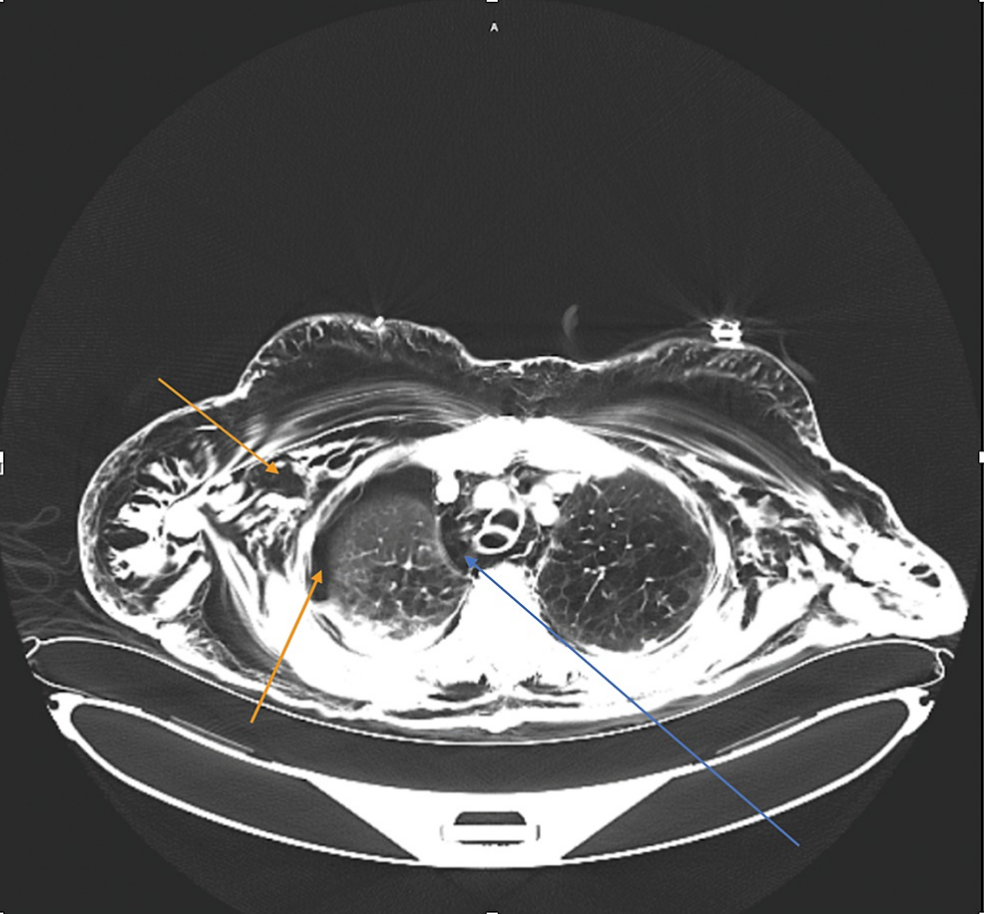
Computed tomography (CT) abdomen/pelvis (A/P) with intravenous contrast - axial view CT A/P in the regular window. Evident is the presence of extensive subcutaneous emphysema (yellow arrow), pneumomediastinum (blue arrow), and a right-sided pneumothorax (orange arrow).
